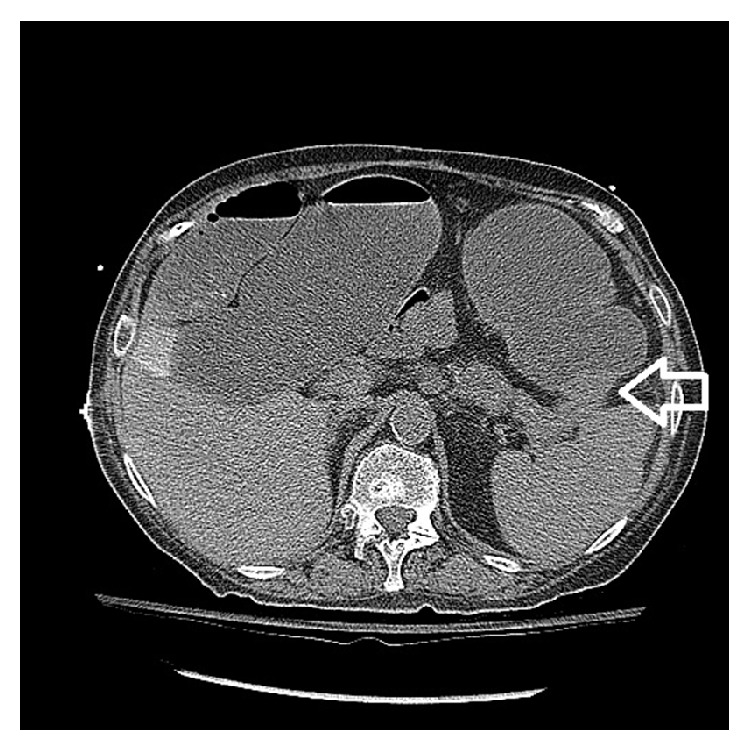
CT abdominal/pelvis obtained after repeat colonoscopy negative for intraluminal obstruction and return of abdominal distention and increase of abdominal pain. Question of pancreatic colonic fistula.
